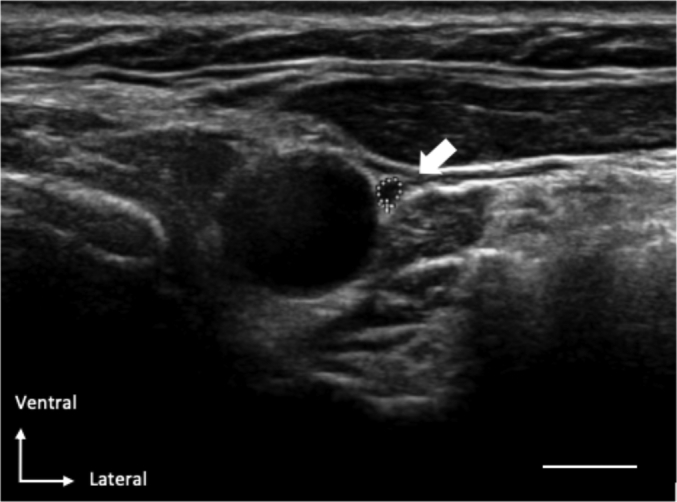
Representative example of an ultrasound image in which the vagus nerve (dotted circle) is positioned ventrolateral to the common carotid artery. The scale bar equals 0.5 cm.
